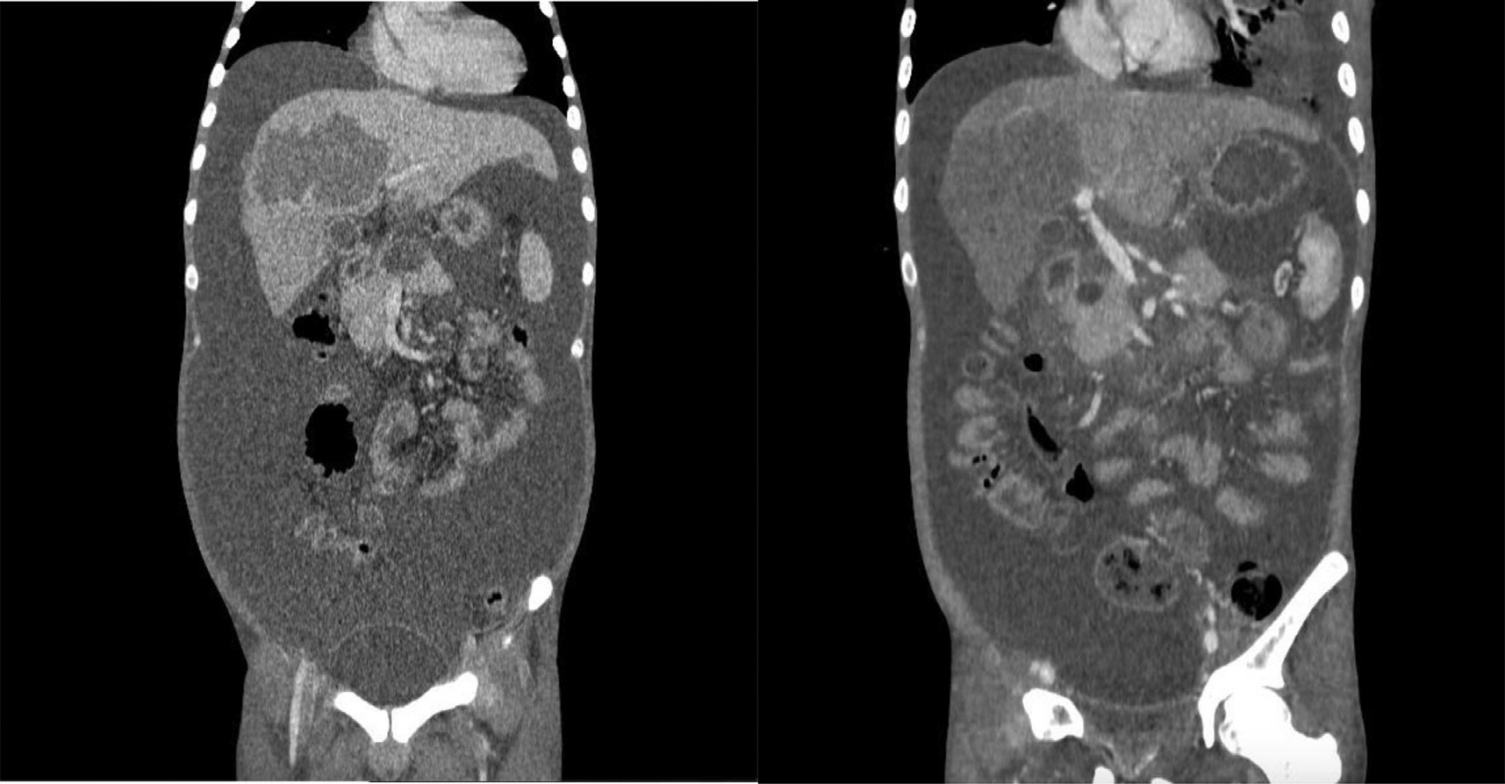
Panel (A) is the liver condition before the course of tuberculosis regimens (November 2021), and panel (B) is the follow-up CT scan after 3 months of treatment (January 2022). Even though the lesions were visible in both pictures, we could appreciate the improvement in the second result.
